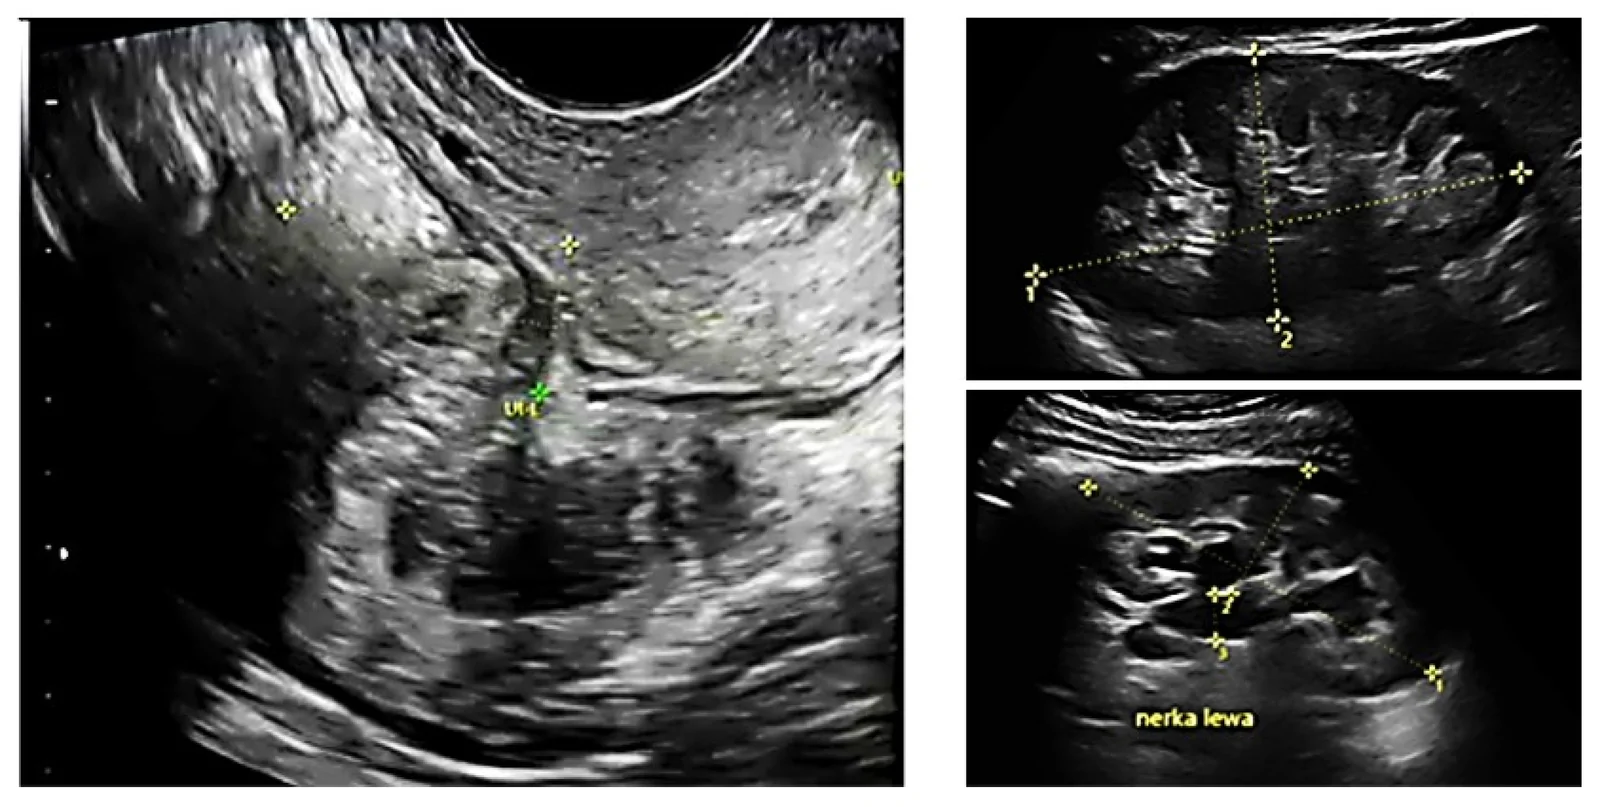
Preoperative ultrasound examination: the bowel DE nodule (TVS exam), congestion in the left kidney (TAS exam).
